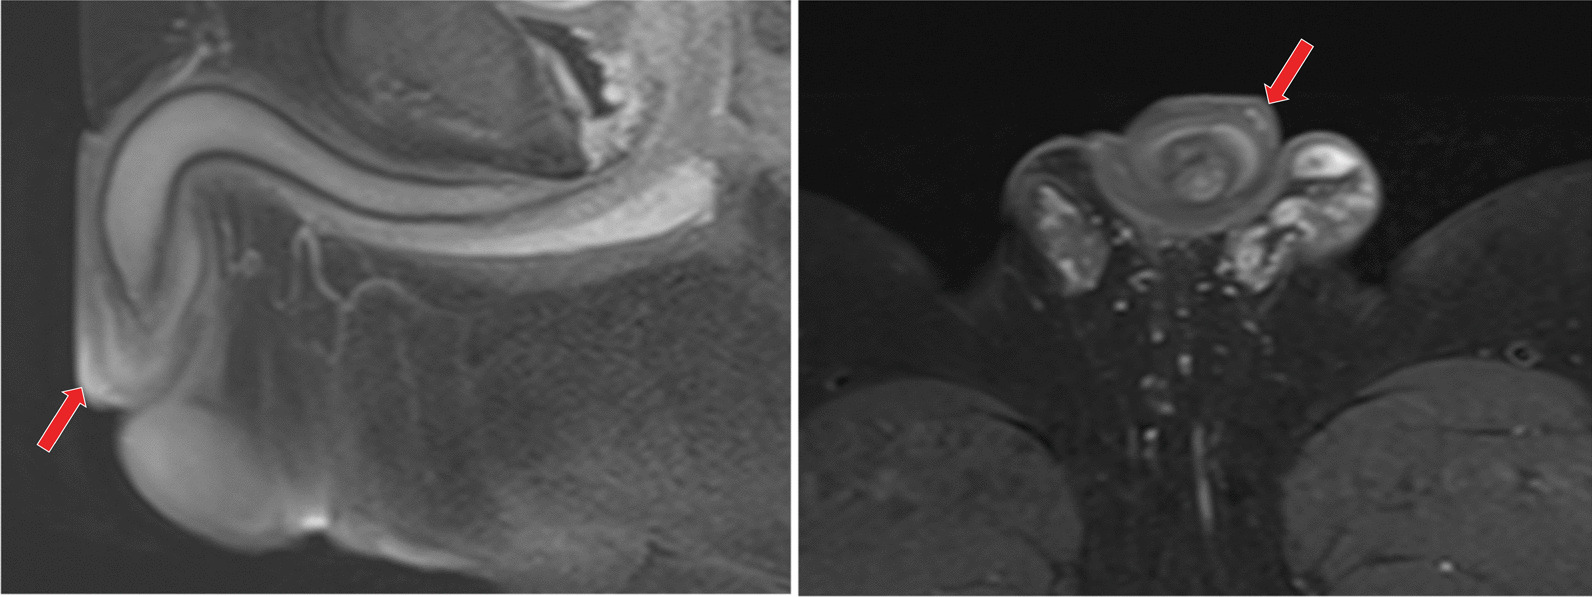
MRI finding—a single hyperintense mass inside glans (arrow), corpora cavernosa are normal, sagittal (A) and coronal (B) cut
